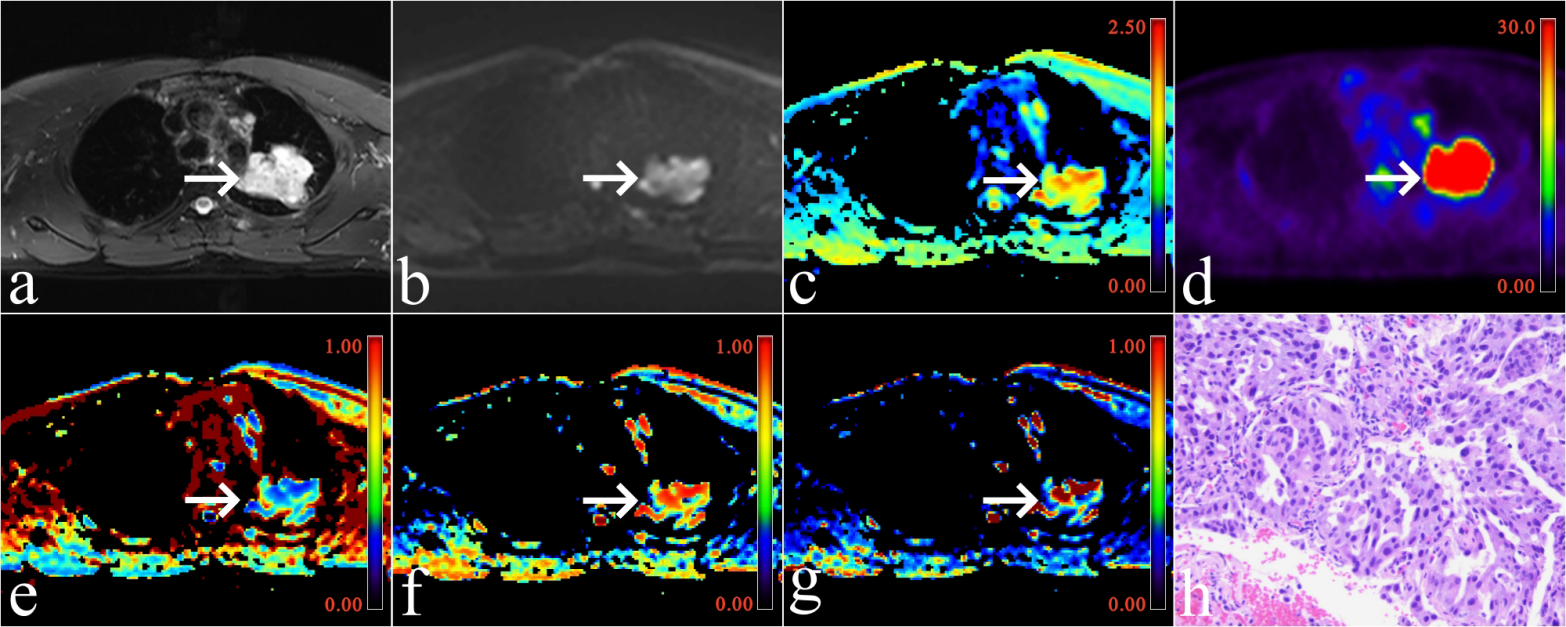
A male patient with adenocarcinoma of the upper lobe of the right lung (arrowheads, maximum diameter = 5.5cm, no smoking). (a) Map of T2-weighted imaging; (b) Map of DWI (b = 600 s/mm^2^); (c) Map of ^18^F-FDG PET; (d) Pseudo colored map of ADC; (e) Pseudo colored map of f_1_; (f) Pseudo colored map of f_2_; (g) Pseudo colored map of f_3_; (h) Pathological images (original magnification, ×100).
